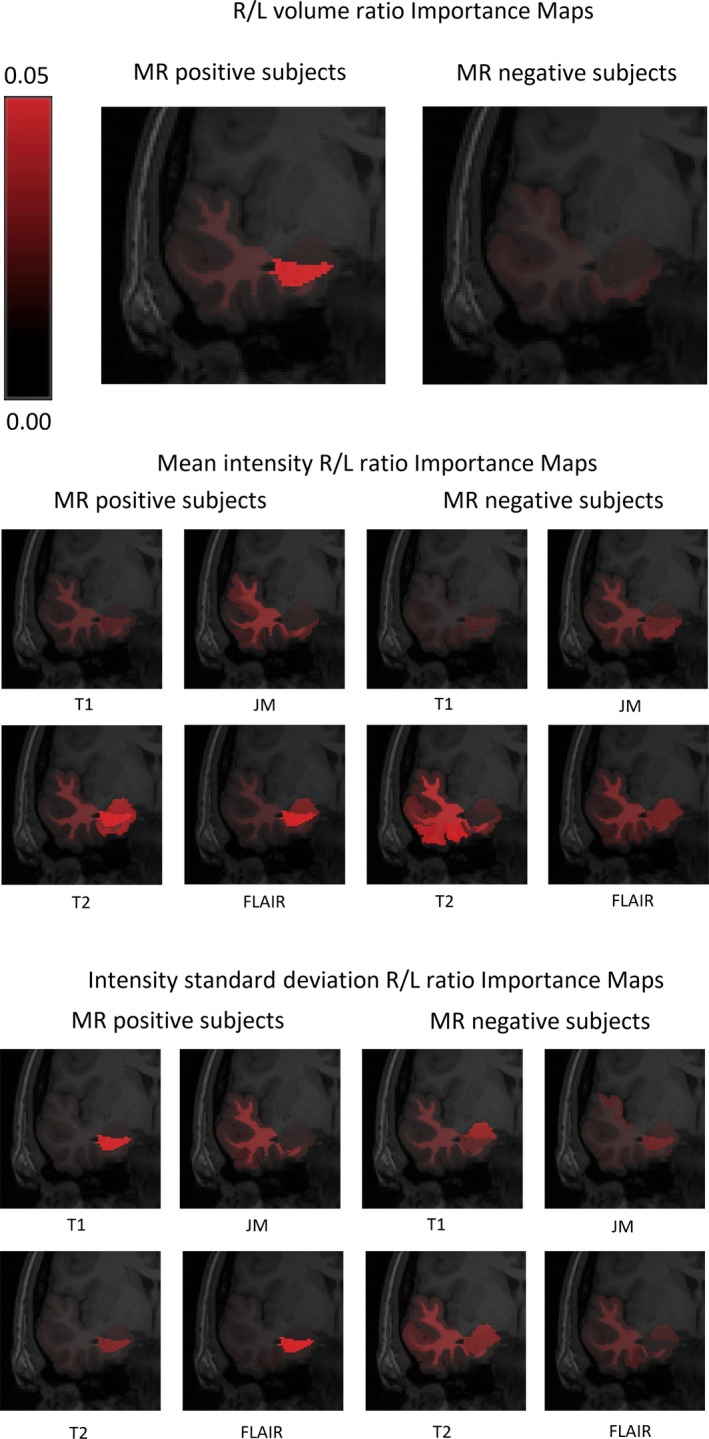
Importance Maps for regional volume asymmetry (top), mean intensity asymmetry (middle), and intensity standard deviation asymmetry (bottom) features in each image type across the temporal lobe for magnetic resonance imaging (MRI)‐positive (left) and MRI‐negative cases (right). The intensity of red within the maps signifies the importance assigned to each region. FLAIR, fluid‐attenuated inversion recovery; JM, junction map; L, left; MR, magnetic resonance; R, right
